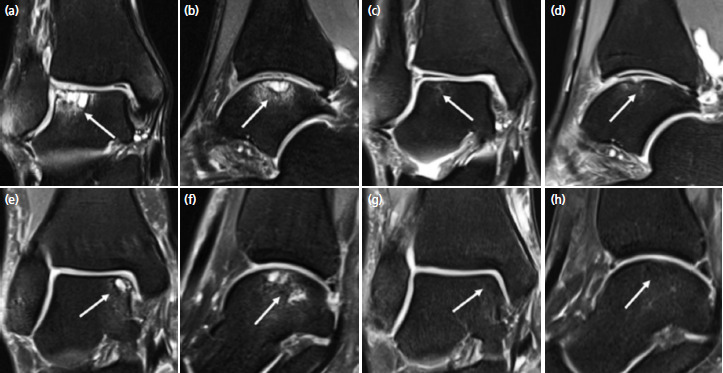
(a) Coronal and (b) sagittal MR images of the second case showing right ankle osteochondral lesion at the lateral aspect of the talar dome (white arrows). Corresponding images at 2 years, (c) and (d) showing osteochondral regeneration and integration (white arrows). MR images of the third case with coronal (e) and (f) sagittal views of the right ankle showing osteochondral lesion at the medial aspect of the talar dome (white arrows). Corresponding images at 2 years, (g) and (h) showing satisfactory healing. These are all fat-suppressed proton density-weighted MR images.
